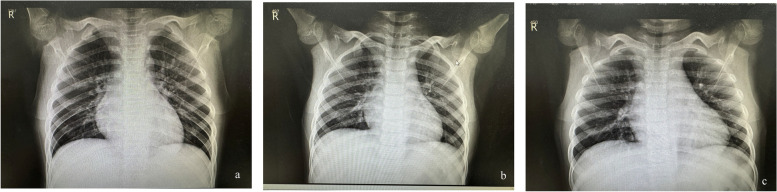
a patient 1. X-ray imaging of bilateral lung texture was disordered, enlarged, and blurred; b patient 2. X-ray imaging bilateral lung texture was disordered and enlarged; c patient 3. X-ray imaging bilateral lung texture was enlarged and blurred
